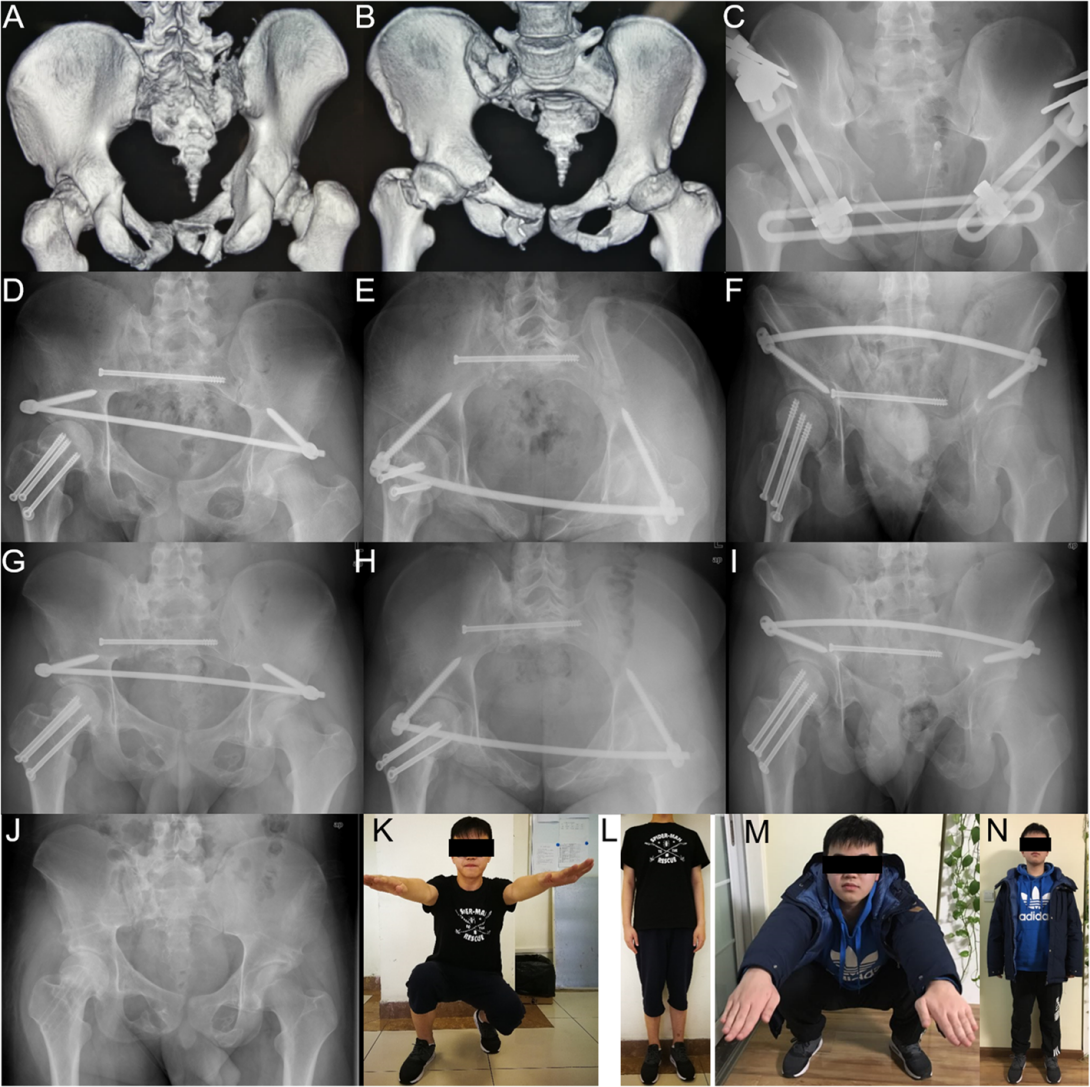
A 17-year-old man with anterior and posterior pelvic ring fractures and right femoral neck fracture. a, b Preoperative 3D CT reconstruction of pelvis. c AP pelvic film after external fixation. d–f Postoperative pelvic films (AP, inlet and outlet views) showing fracture union at 6 months. g–i Postoperative pelvic films (AP, inlet and outlet views) at 12 months. j AP pelvic film after implant removal at 12 months. k, l Walking and standing were not limited while right hip flexion was limited at 6 months follow-up. m, n Hip Flexion was more than 90°and Majeed score was excellent at 12 months follow-up
